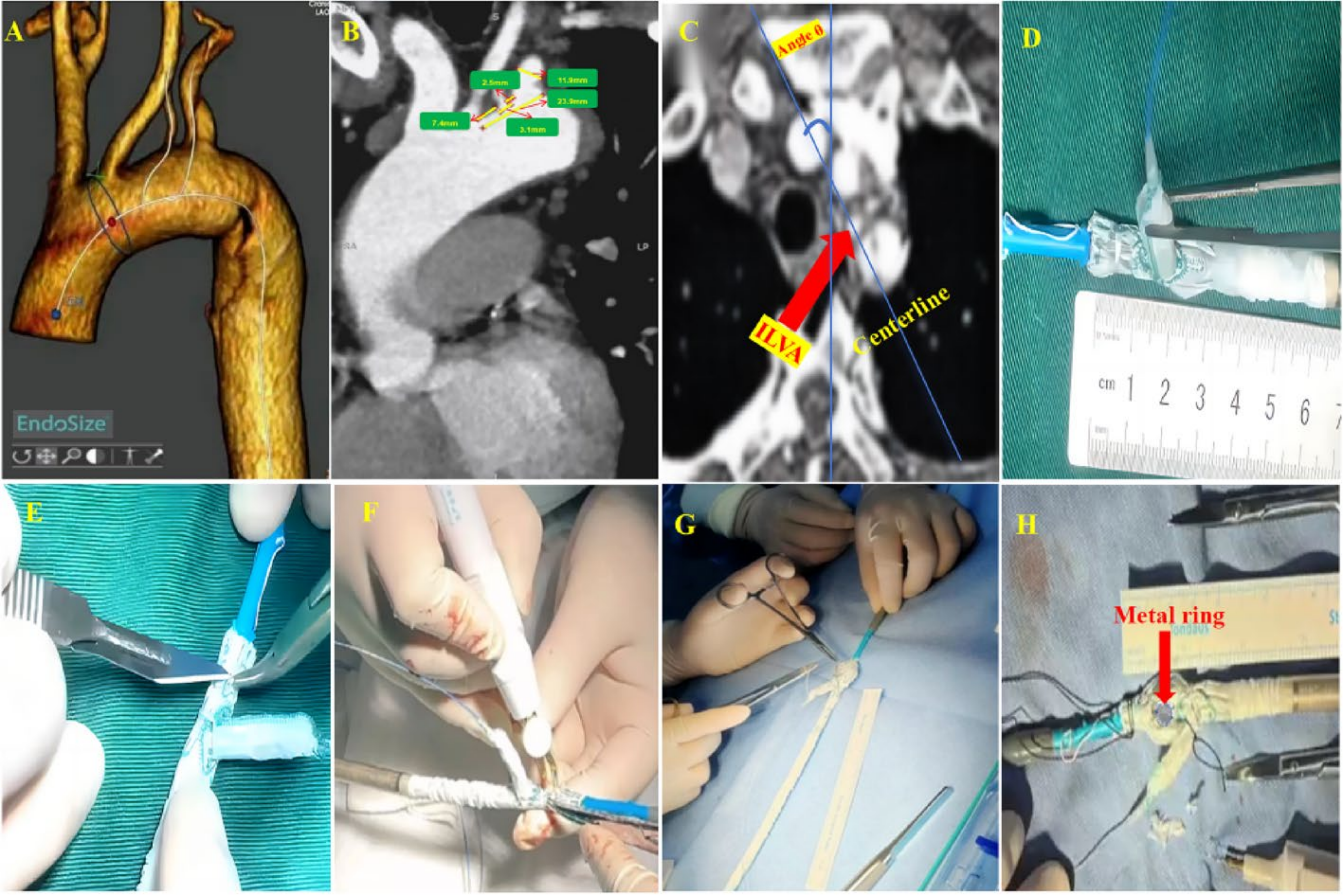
(A–C) Accurate measurements of the import parameters based on the CTA using EndoSize software before the PMF of ILVA. (D–H) The PMF of ILVA was performed on the Castor main stent. *CTA* computed tomography angiography, *PMF* physician modified fenestration, *ILVA* isolated left vertebral artery.
